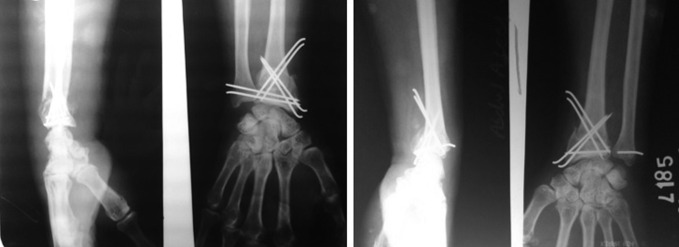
Radiograph showing pin breaking.
